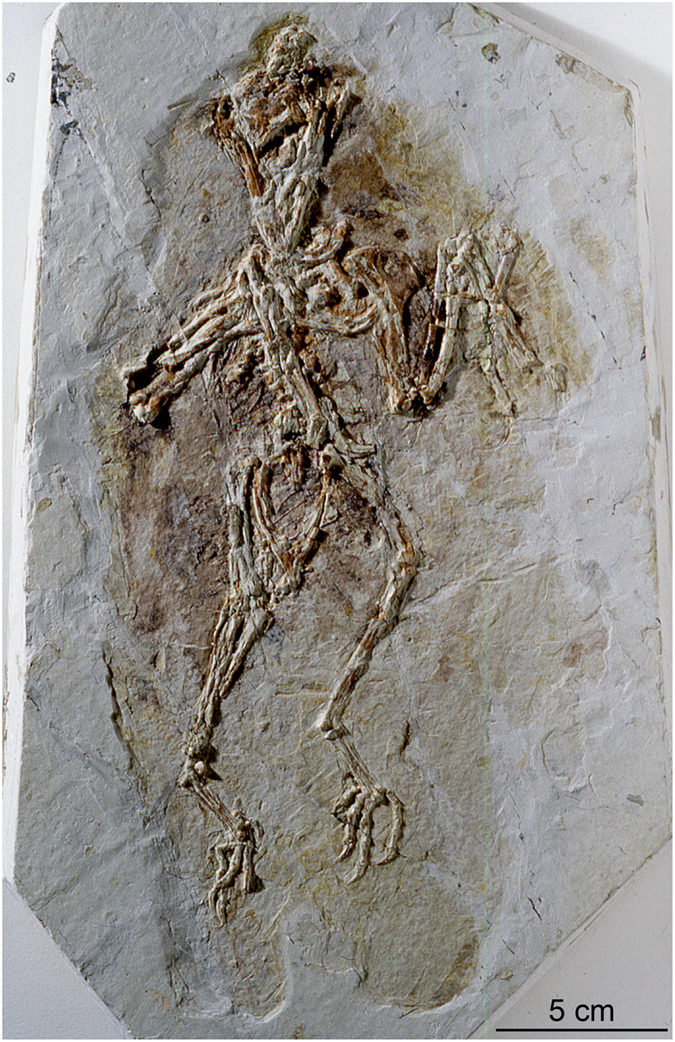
Photograph of the *Confuciusornis* amalgamation as it was presented for CT scanning in 1998, taken with 35 mm color slide film (Photography by Joe Jaworowski).
